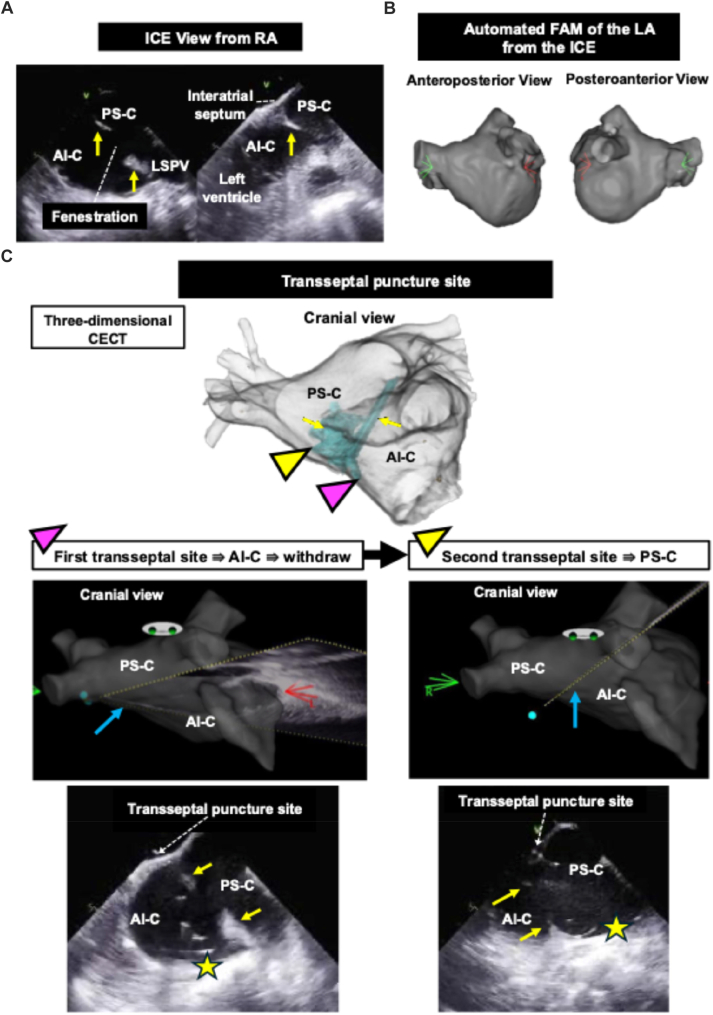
ICE and 3-dimensional imaging of cor triatriatum sinister. A: ICE from the right atrium showing the left atrial membrane attached to the anteroinferior fossa ovalis, forming a fenestration between the AI-C and the PS-C. B: Automated FAM of the LA generated from ICE. C: Transseptal puncture guided by ICE and 3-dimensional computed tomography. The first puncture entered the AI-C, whereas the second, targeted above the membrane, successfully accessed the PS-C. *Yellow arrows*: the LA membrane. *Yellow star*: Versacross radiofrequency wire. *Blue arrows*: the direction and position of the ICE imaging beam in the 3-dimensional mapping system. *Pink triangle*: first transseptal puncture site. *Yellow triangle*: second transseptal puncture site. AI-C = anteroinferior compartment; CECT = contrast-enhanced computed tomography; FAM = fast anatomic map; ICE = intracardiac echocardiography; LA = left atrium; LSPV = left superior pulmonary vein; PS-C = posterosuperior compartment; RA = right atrium.
